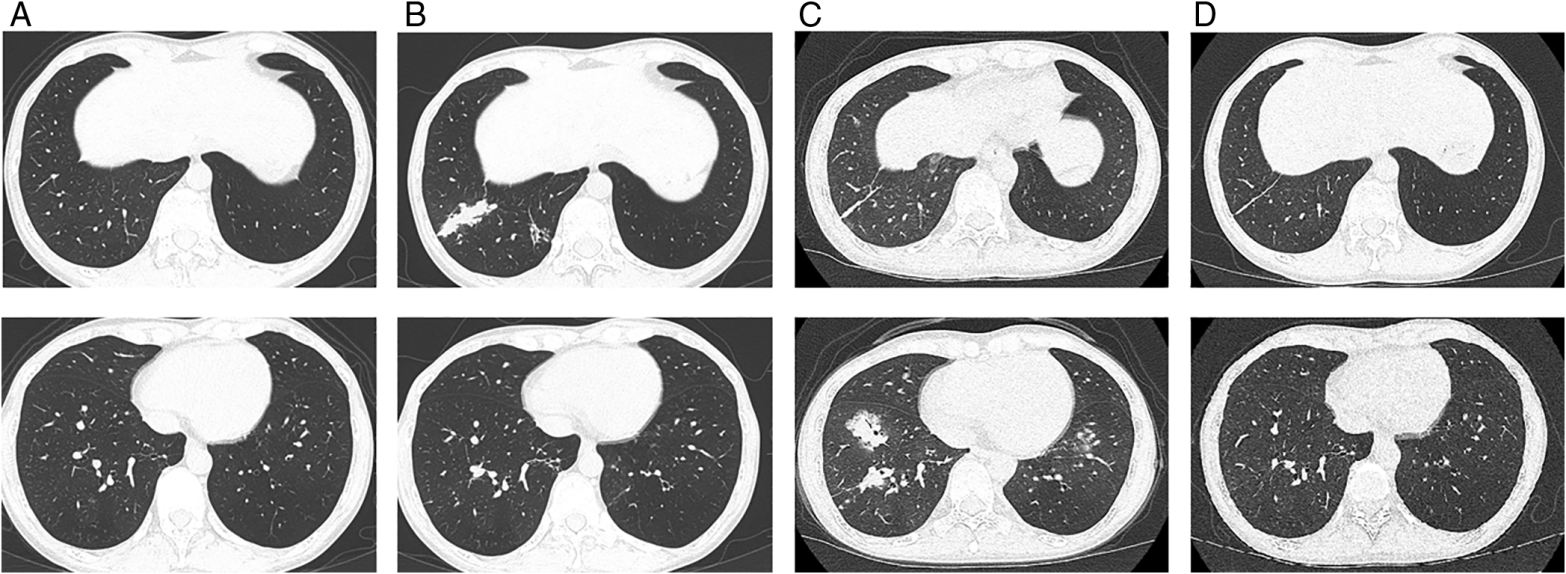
Chest computed tomography images before and after bronchial thermoplasty (BT). The images in the upper section are slices around the right segment B9, and the images in the lower section are slices around right lower lobe segments (A) before BT and after BT completion at (B) 1 month, (C) 7 months, and (D) 12 months.
